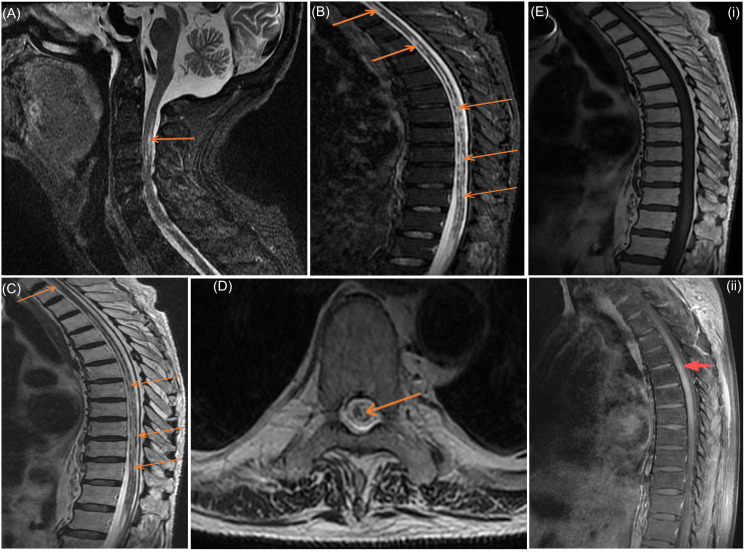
Spine MRI findings. (A–C) Sagittal cervical and thoracic MRI showed longitudinally extensive intramedullary lesions from C1 to C5 and T2 to T12, without vertebral involvement or spinal cord compression. (D) Axial T2-weighted imaging showed patchy intramedullary hyperintensity. (E) Pre-contrast sagittal T1-weighted imaging (T1WI) showed no definite enhancement (i), whereas contrast-enhanced sagittal T1WI showed linear peripheral spinal cord enhancement (ii).
